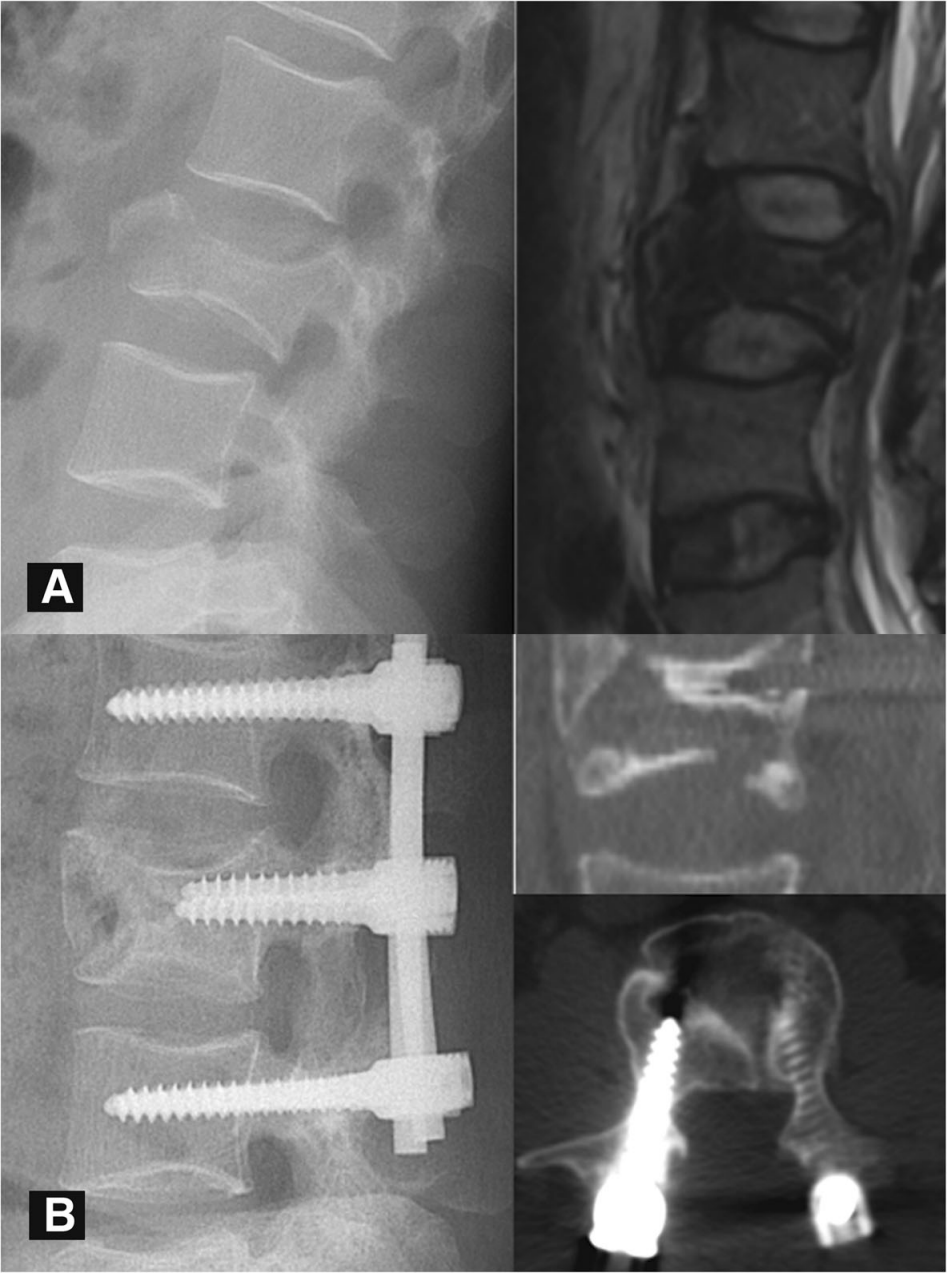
A 41-year-old male had a burst fracture at L3, with an ASIA grade for neurological function of C on admission. a Preoperative X-ray and MR images show an A4 burst fracture. The fracture mass protruded into the spinal canal and compressed the dura mater. b X-ray and CT images 18 months after surgery. The collapsed vertebra was well reduced and maintained. Several burst fragments converged into a cavity within the vertebral body, violating both endplates and the anterior vertebral shell, which was classified as HPC type IV
